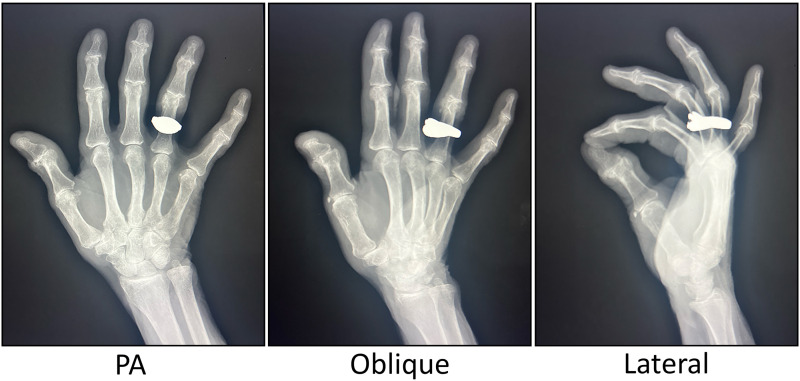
Radiographs of the right hand from September 2023 demonstrating moderate osteoarthritis in the right thumb CMC joint. CMC, carpometacarpal; PA, posteroanterior.
